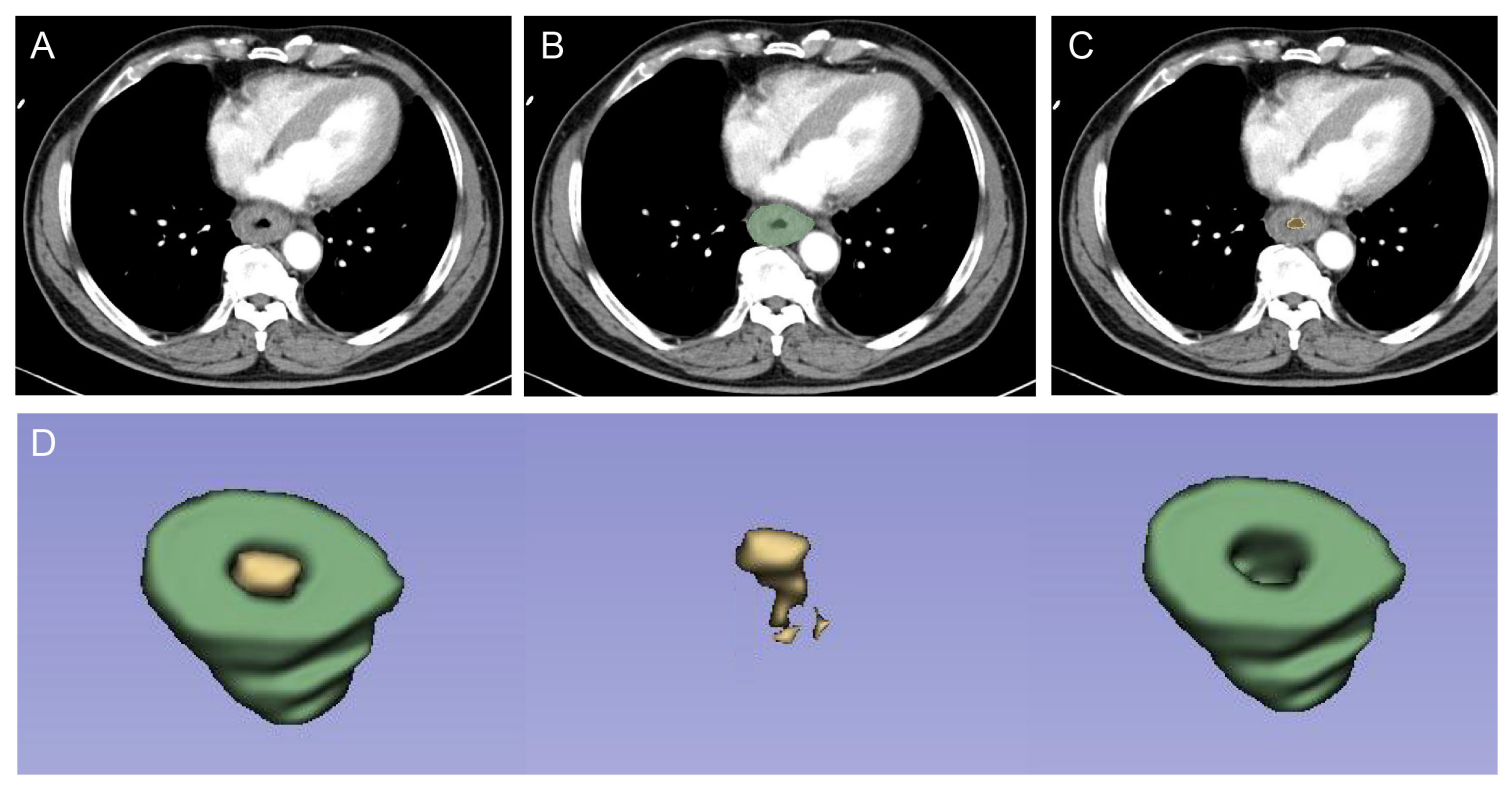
ROI segmentation for radiomic analysis. First, radiologists checked the lesion on contrast-enhanced CT at the arterial phase (A). Then, the GTV (B) and intra-luminal air from GTV (C) were drawn, respectively. Finally, the computer automatically segmented the two regions, which resulted in the identification of the volumetric features of the tumor (ROI, green) and intra-luminal air (yellow) (D). ROI was used for subsequent radiomics feature extraction and model construction. ROI, region of interest; GTV, gross tumor volume.
